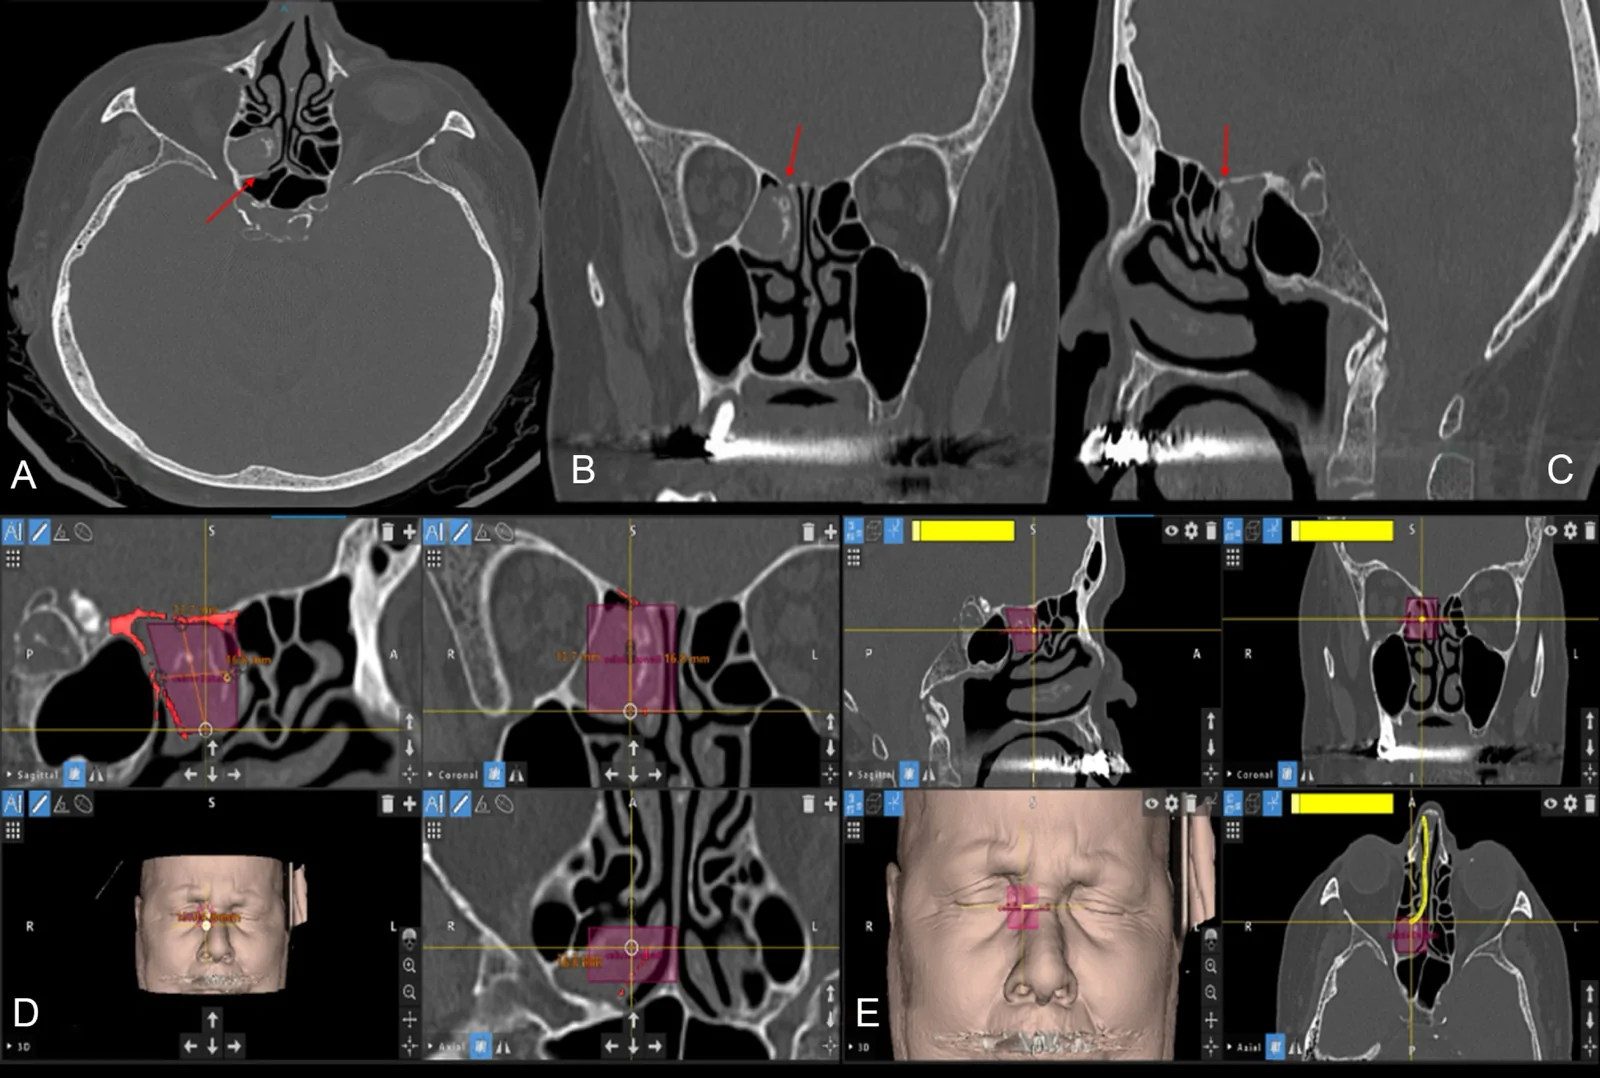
(a–c) Preoperative CT scan images (axial, coronal, and sagittal) showed total opacification of right Onodi cell with multiple calcifications and areas of bony lysis. (d and e) Preoperative 3D planification of right Onodi cell with AR navigation system.
